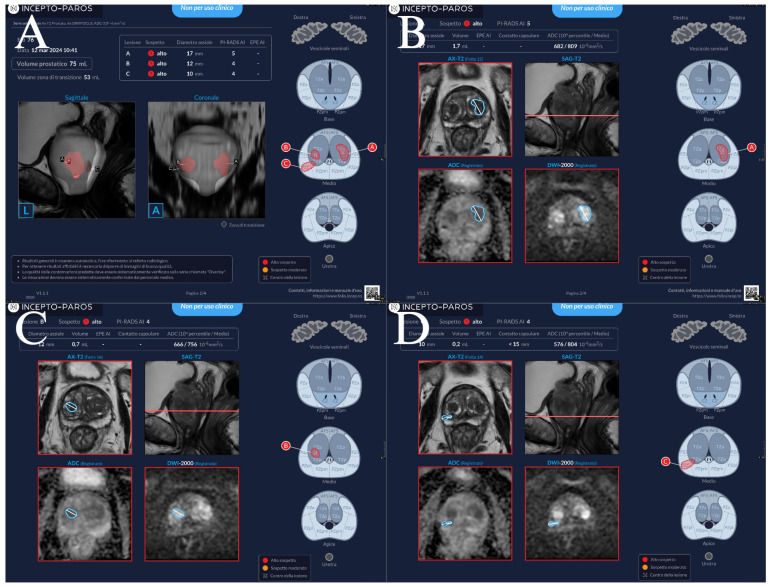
False positive prostate MRI reported by AI-powered tool (A). In this prostate MRI, the AI identified one PI-RADS lesion with a score of 5 in the transition zone (B), and two PI-RADS lesions with a score of 4 in the transition (C) and in the peripheral zone (D). The radiologist did not report any lesion with a PI-RADS score of 3 or higher. At biopsy, no prostate cancer was found. Clinical implication: this example highlights the potential risk of AI-driven overestimation of lesion suspicion, which could increase unnecessary biopsy if AI outputs are used without expert radiologist verification.
